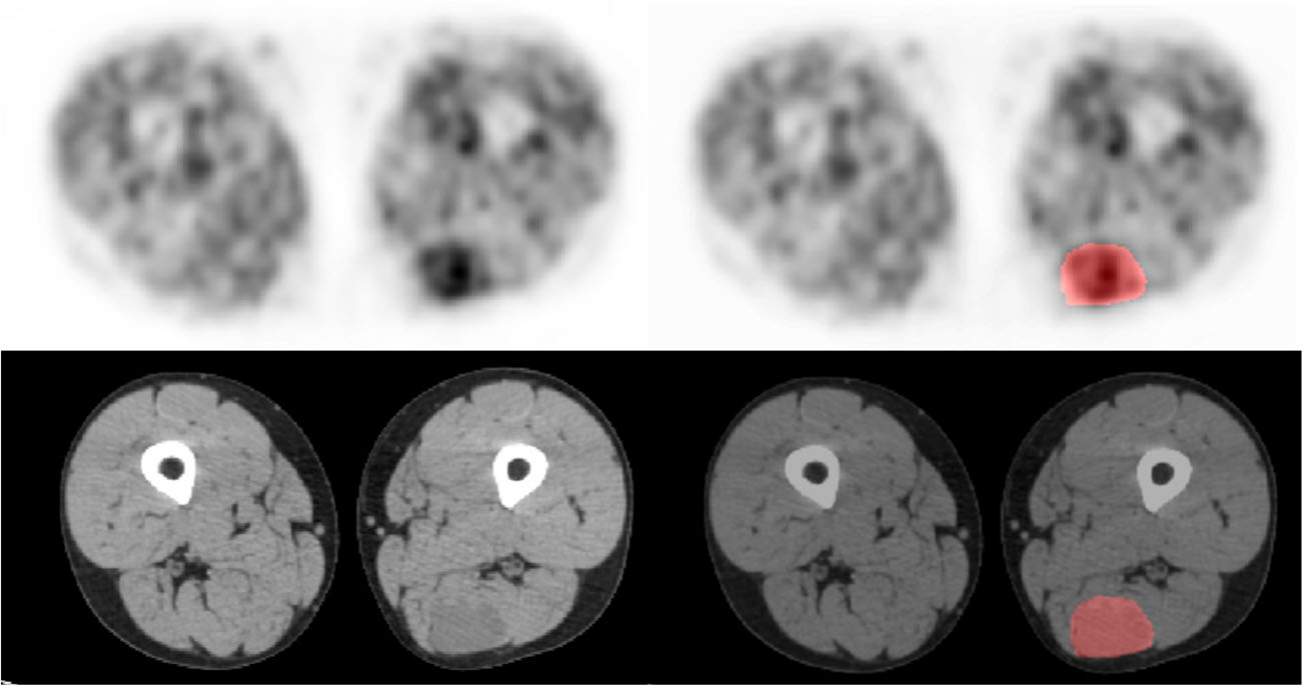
^18^F-FDG PET and CT (*left*) with corresponding images with ROIs (*right*). A symptomatic but benign left posterior thigh neurofibroma (SUVmax = 2.83)
